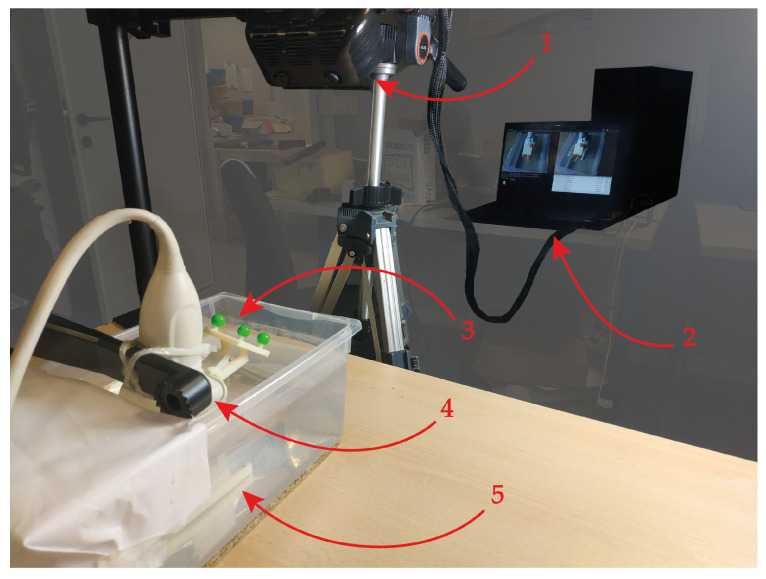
Set-up implemented for the calibration: 1 → The augmented reality head-mounted display employed. 2 → The laptop running the RGB tracking algorithm. 3 → The optical frame anchored to the ultrasound probe. 4 → The mounting arm holding the ultrasound probe, which enables it to be moved in space. 5 → The calibration comb dipped in the 37° water bath.
